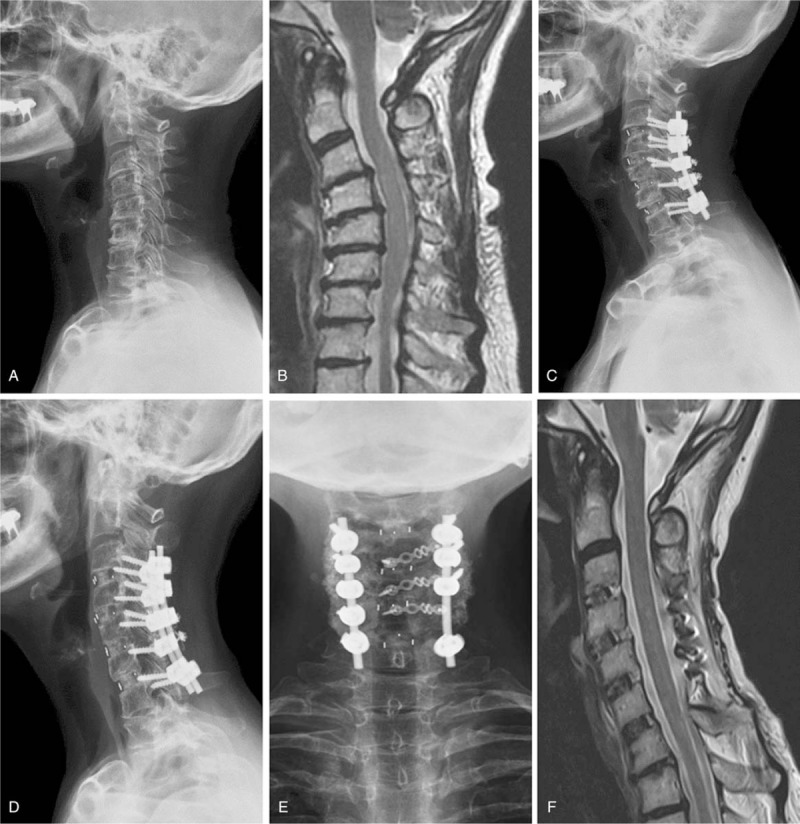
A 66-year-old woman received C3-7 ACDF with PEEK cages, PIF with C3-6 lateral mass screws and C7 pedicle screws, and PD with C3-6 laminoplasty. (A) The preoperative plain film demonstrated 13° cervical kyphosis. (B) Midsagittal T2-weighted MRI revealed spinal cord compression over C3-7 kyphotic stenosis. (C) The postoperative 3-month plain film revealed restoration of the cervical lordotic curve (18° lordosis) with augmented PIF and a chipped bone graft placed over the laminae of the hinge side and bilateral mass. (D, E) The plain films 12 months postsurgery reveal union over the anterior fusion segments and posterior bilateral mass. (F) Midsagittal T2-weighted MRI 12 months postsurgery reveals patent spinal canal over C3-7. ACDF = anterior cervical discectomy fusion, MRI = magnetic resonance imaging, PEEK = polyetheretherketone, PIF = posterior instrumented fusion.
